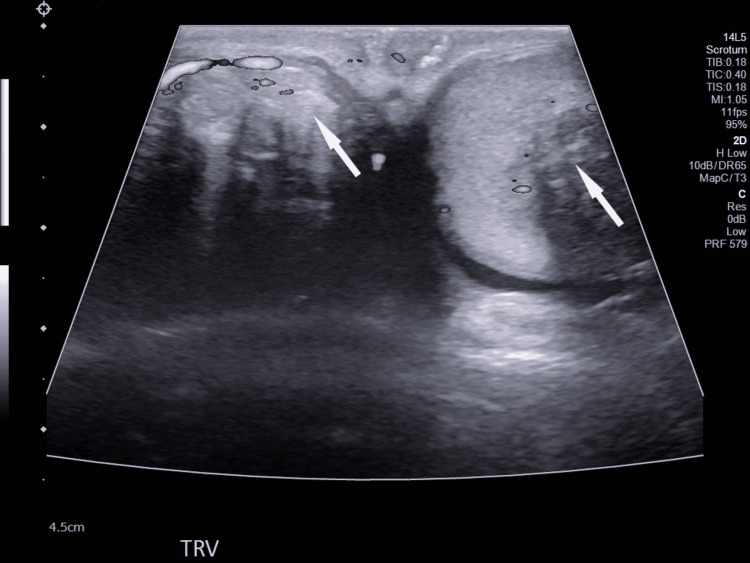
Color duplex transverse ultrasound image of the bilateral testicles demonstrating multiple, heterogeneous, shadowing bilateral testicular masses (white arrows).
